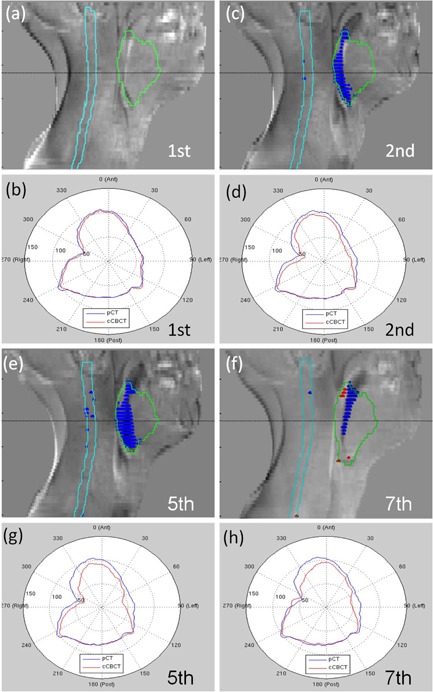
PDR differences and polar WET plots ((a) to (h)) of cCBCT relative to pCT for week 1, 2, 5, and 7. The GTV and spinal cord contours are projected in green and cyan respectively. Blue and red regions indicate points with an accumulated WET difference greater than 3 mm within a 3 mm radius.
